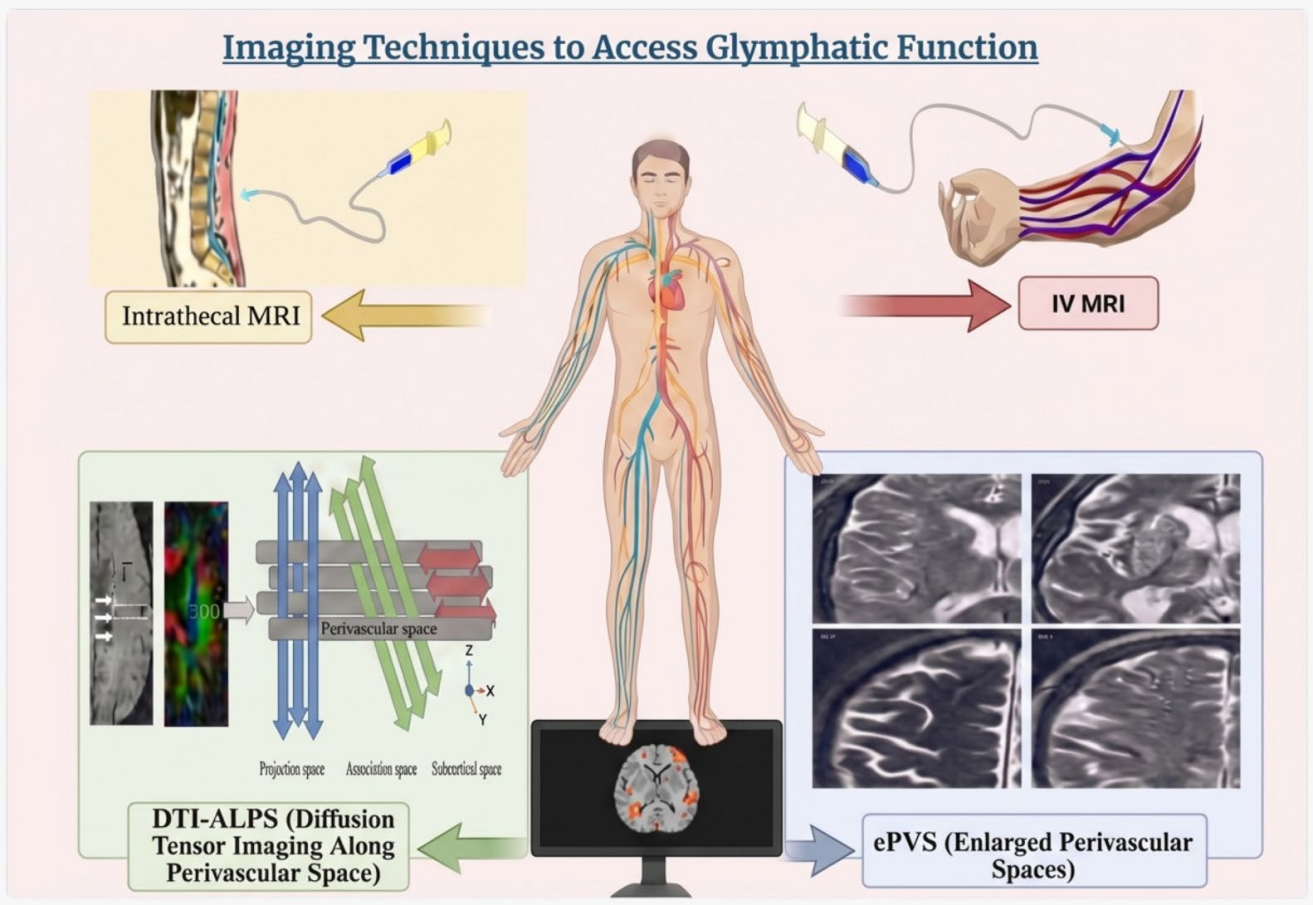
Imaging techniques to assess glymphatic function. A range of imaging modalities is used to evaluate glymphatic activity. Intrathecal and intravenous contrast‐enhanced MRI visualizes CSF flow; DTI‐ALPS quantifies directional water diffusion along perivascular spaces; and enlarged perivascular spaces (ePVS) serve as structural biomarkers of impaired clearance. Created in https://BioRender.com.
